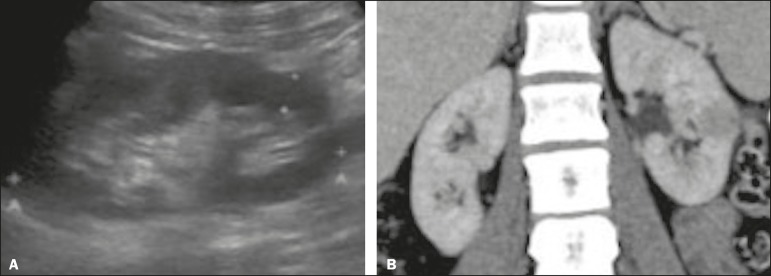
Focal pyelonephritis. Ultrasound (A) and CT (B) of the left kidney
